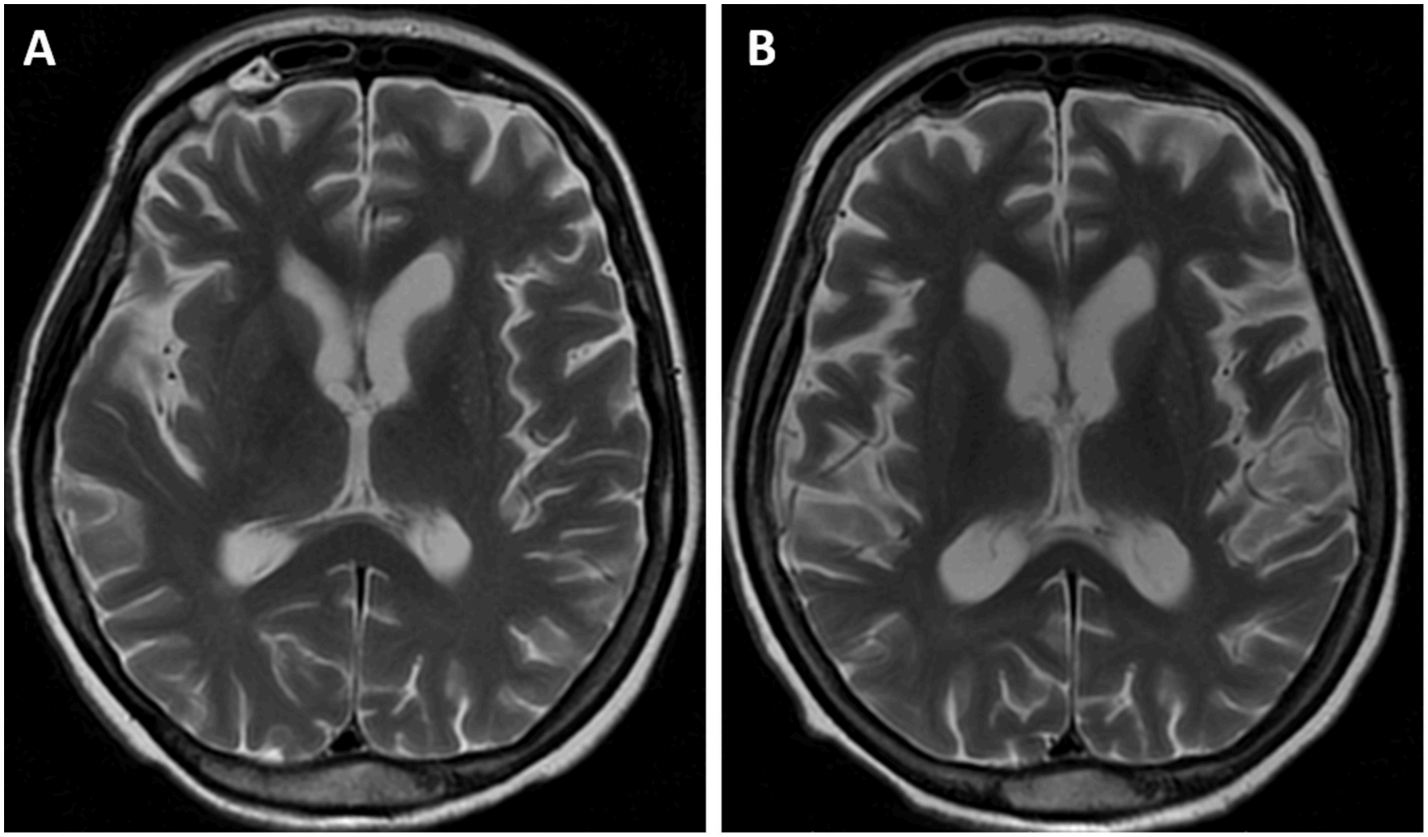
MR examination, axial T2-weighted images. The initial MR showed diffuse, mild cortico-subcortical atrophy of the brain (A). The follow-up image (B) revealed an increased rate of brain atrophy after 2 years.
